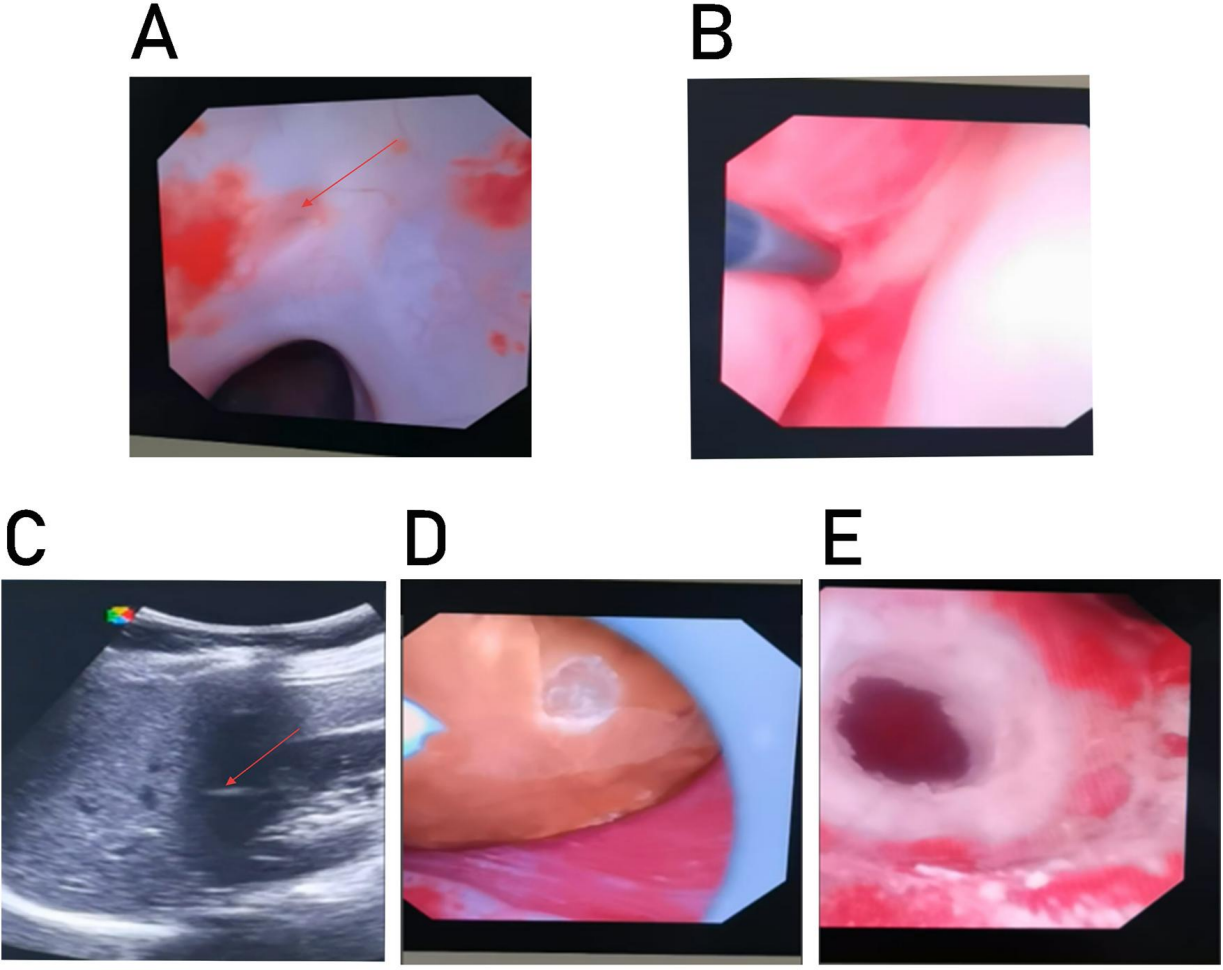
(A) Suspected non-communicating diverticulum orifice. (B) The resistance at the tip of the guidewire decreases sharply into the cavity. (C) Ultrasound positioning. (D) Yellow-brown oval stones were found. (E) Circumferential incision of the diverticulum opening.
